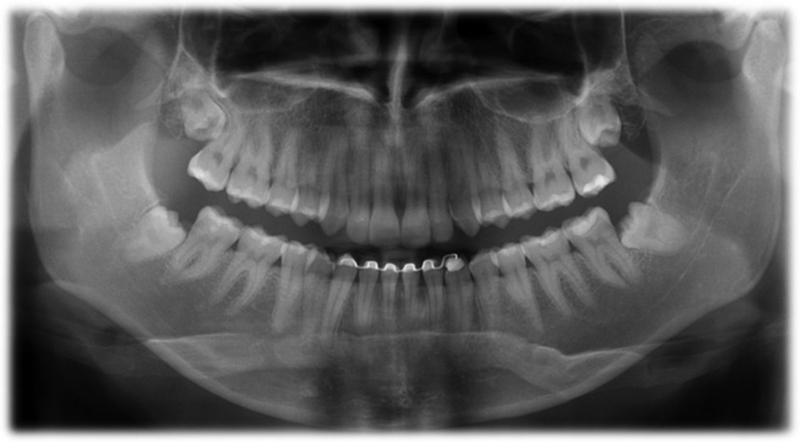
Panoramic radiography, third molar retained.
